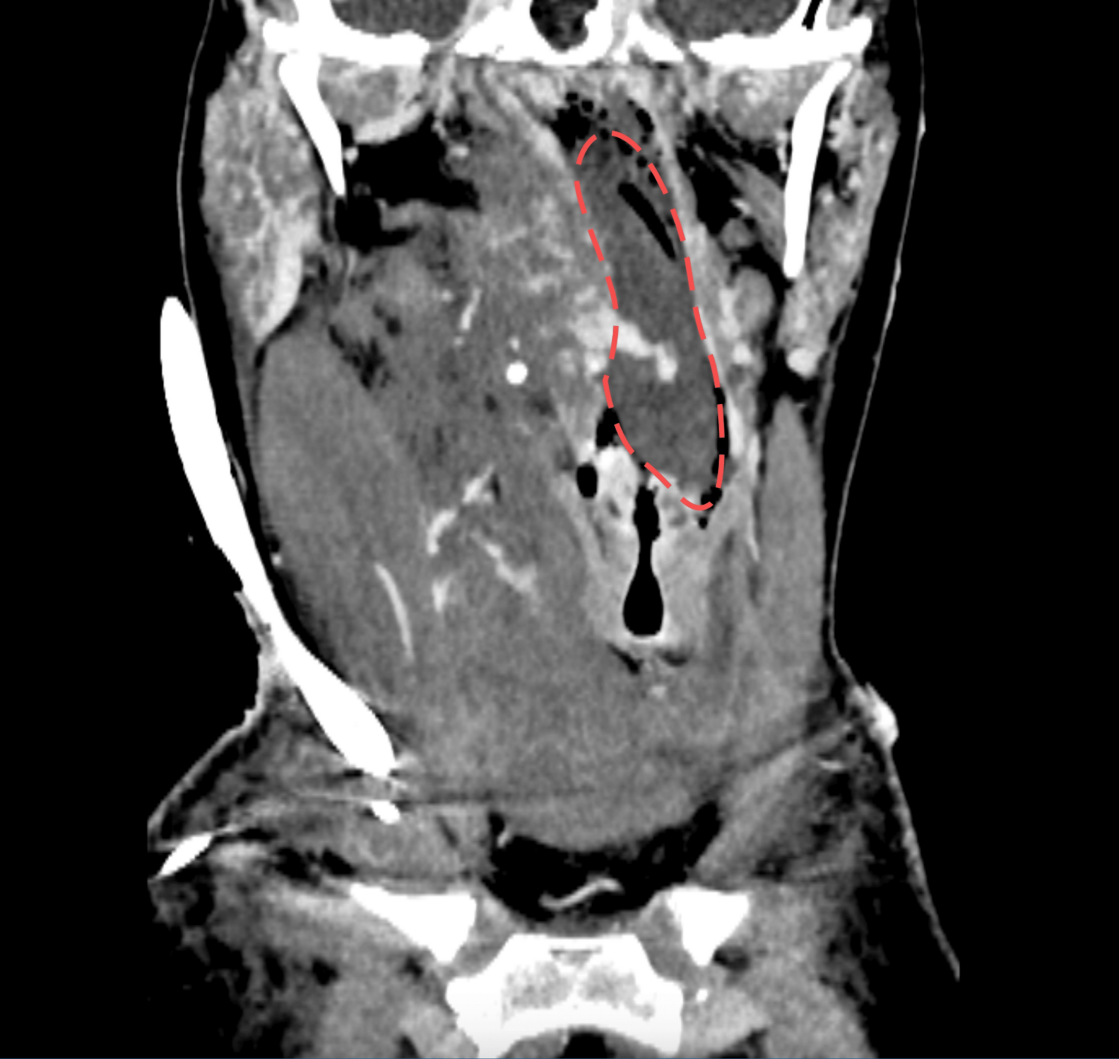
The coronal CT scan Unclear mass in throat marked with red lines.
